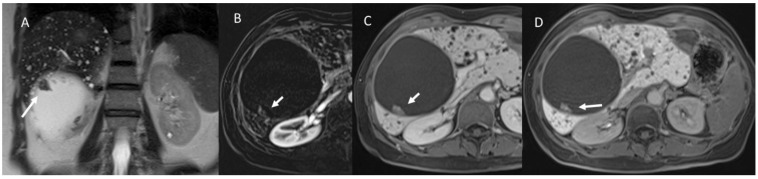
MRI assessment of female cystadenocarcinoma patient. The white arrows show papillary soft tissue growths inside cystic lesion with solid appearance in T2-weigthed (A) and progressive contrast enhancement during arterial (B) (subtract phase), portal (C), and liver-specific phase (D) using T1-weigthed post contrast sequences (volumetric interpolated breath-holding examination sequence).
